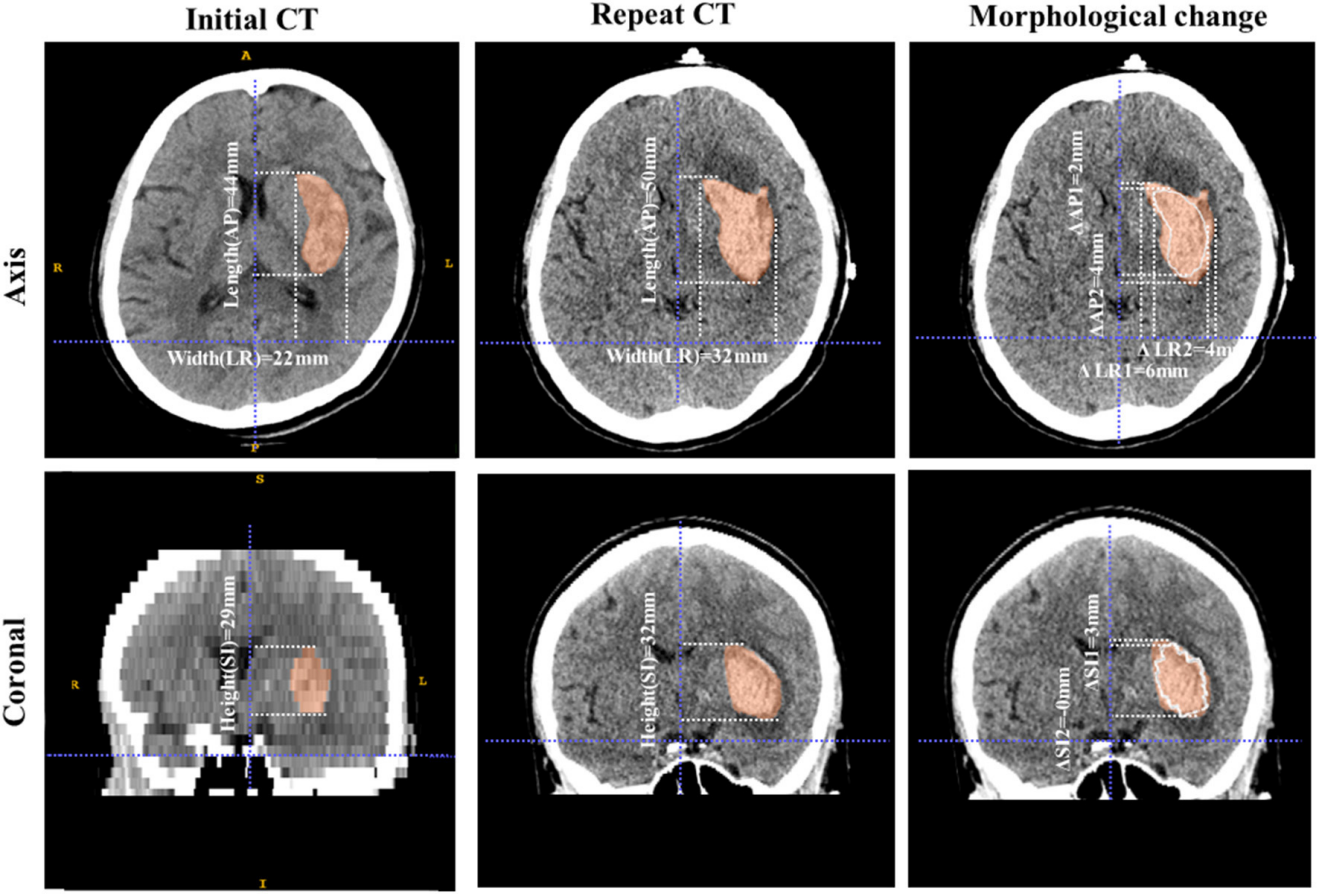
Example of morphological change in a hematoma. These images are from a 73-year-old male patient with ICH. The volume of the hematoma was 15.6 mL on initial CT and 23.5 mL on repeat CT. The first column shows the shape characteristics of the initial hematoma, including its three diameters (length 44 mm, width 22 mm, and height 29 mm). The longitudinal axis is of the AP type and the SR index is 0.628. The second column shows the shape characteristics of the hematoma on the repeat scan, including its three diameters (length 50 mm, width 32 mm, and height 32 mm). The longitudinal axis is of the AP type and the SR index is 0.552. The third column shows the morphological change. The white line is the contour of the initial hematoma and the red area is the hematoma as of the repeat scan. The length changes of the hematoma diameters are 6 mm, 10 mm, and 3 mm in the AP, LR, and SI directions, respectively. The direction change of the hematoma diameters is LR and the longitudinal axis type does not change (AP). The SR index decreases, which means that the hematoma becomes more irregular from initial CT to repeat CT.
